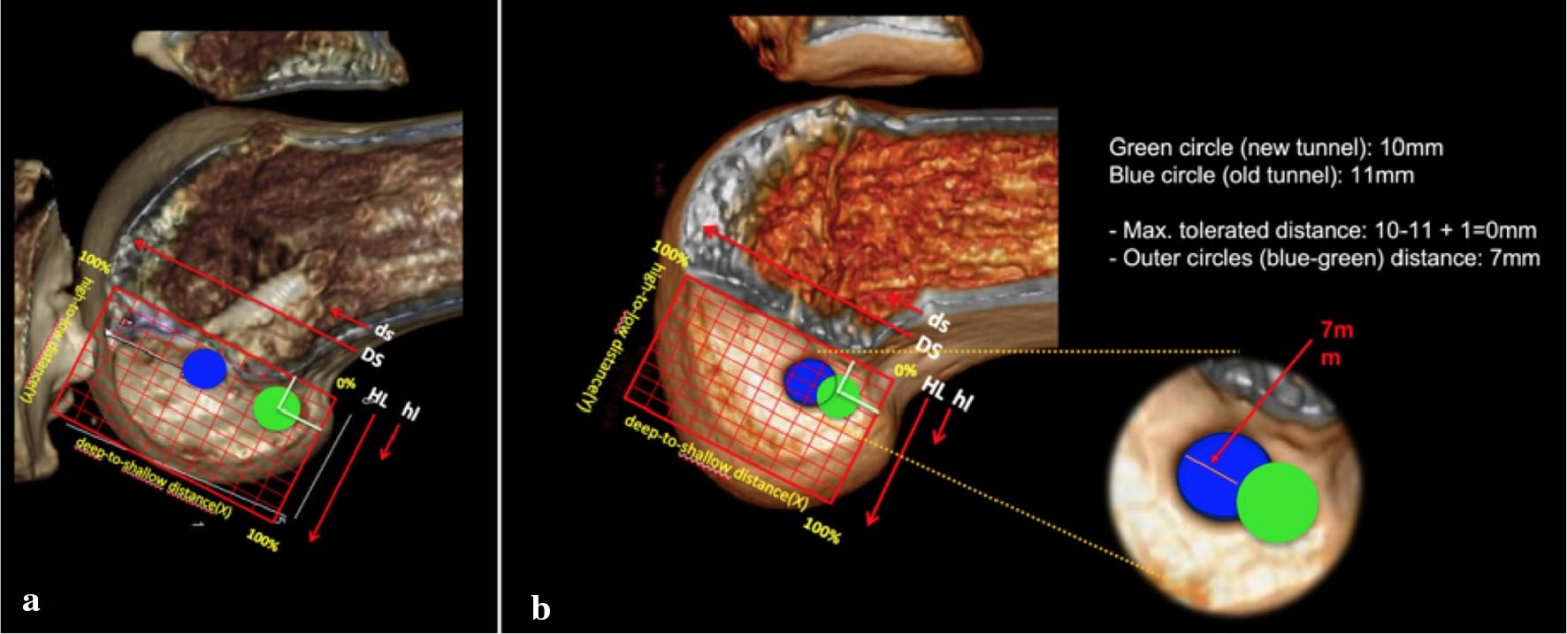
A 3D-CT reconstruction of femoral lateral condyle in the sagittal view (with suppression of the medial femoral condyle), with no superimposition between the previous (blu) and the new planned tunnels (green) B 3D-CT reconstruction of femoral lateral condyle in the sagittal view, case of not tolerated superimposition between the old and new planned tunnels, that required two-stage revACLR (colour figure online)
